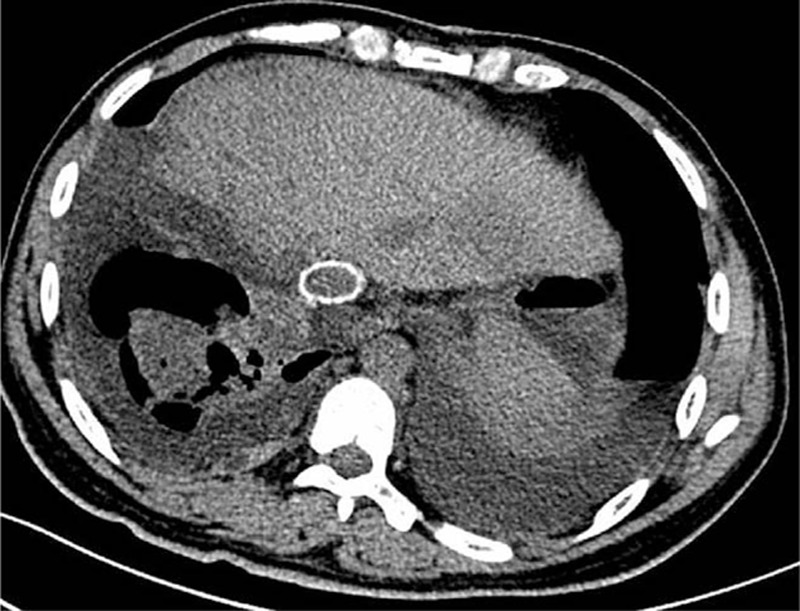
The CT scan at 15 days after the surgery indicated a patent IVC with no obstruction.
